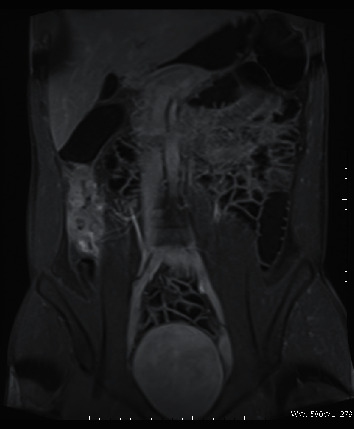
Coronal t1-weighted MRI image with the gadolinium contrast agent, presenting wall thickening and enhancement of the caecum and proximal ascending colon.
